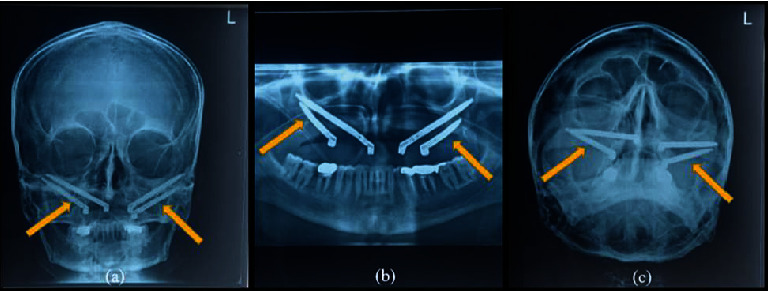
Postsurgical radiographs of the patient: (a) anteroposterior view; (b) orthopantomogram; (c) submentovertex radiographs showing zygomatic implants in place.
